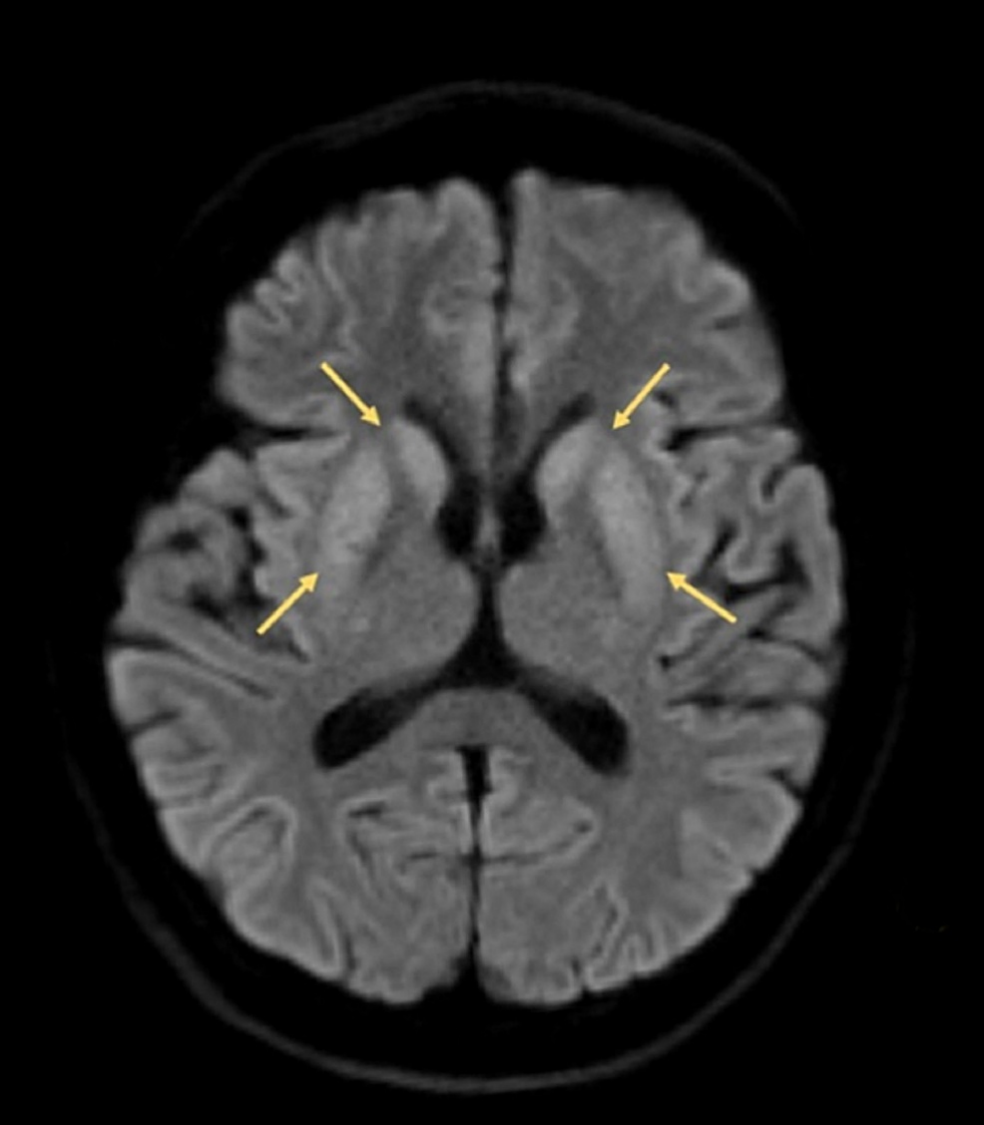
Brain MRI (DWI) on day two of hospital admission. The axial view of the DWI shows symmetrical high-intensity lesions at the bilateral head of caudate nucleus and putamen. DWI: diffusion-weighted imaging
